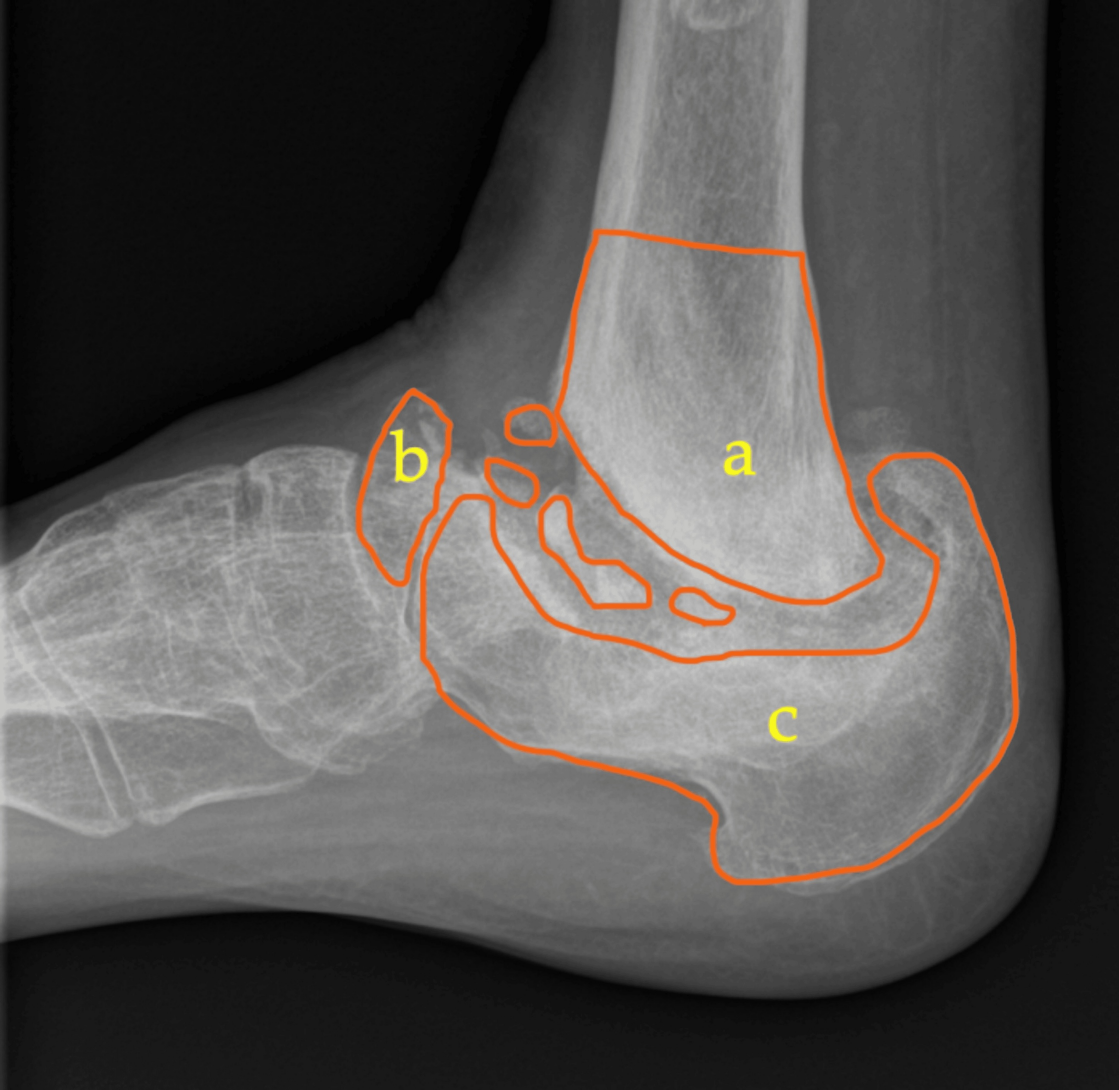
Radiograph depicting the anatomical sites of potential bone loss in ankle Charcot a: distal tibia; b: talus; c: calcaneum The extent of osseous loss may vary based on the chronicity of the condition.
